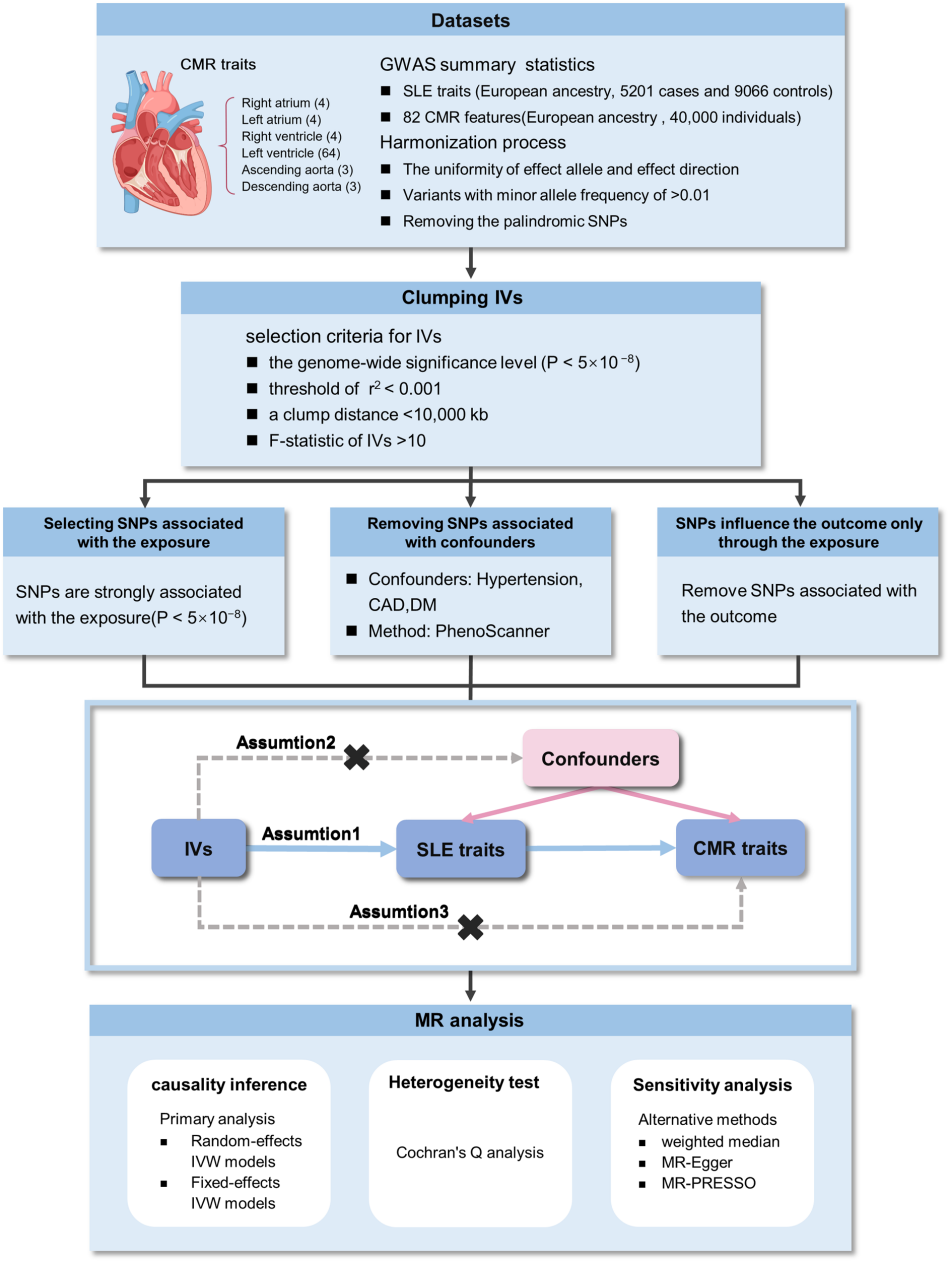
Overview of the study design and analyses. CMR, cardiovascular magnetic resonance imaging; GWAS, genome-wide association studies; SLE, systemic lupus erythematosus; SNPs, single nucleotide polymorphisms; IVW, inverse-variance weighted; IVs, instrumental variables.
